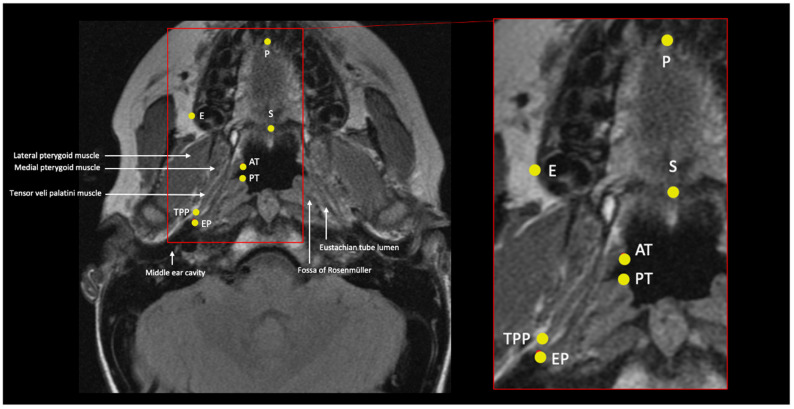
Oblique axial slice from an MRI scan depicting prosthion (P), staphylion(S), ectomolare (E), anterior and posterior torus tubarius (AT and PT), proximal insertion of TVP (TPP), and Eustachian point (EP). This is not the optimal slice for all these landmarks but is included because it demonstrates their relative positions.
